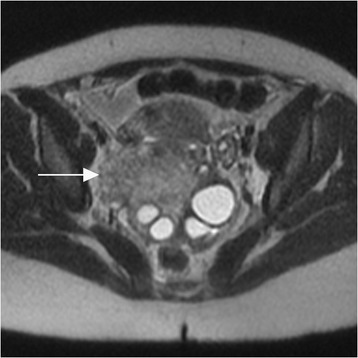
Stimulated ovary without torsion diagnosed as torsion by MRI. Axial T2 weighted MR image of the pelvis in a 31-year-old woman with abdominal pain and leukocytosis at 5 weeks 6 days gestational age demonstrates marked asymmetric enlargement of the right ovary (arrow) with increased right ovarian stromal signal intensity. Prospective and retrospective MRI diagnosis was ovarian torsion, but further history (not available at the time of image interpretation) revealed known asymmetric enlargement of the right ovary since the time of egg retrieval
